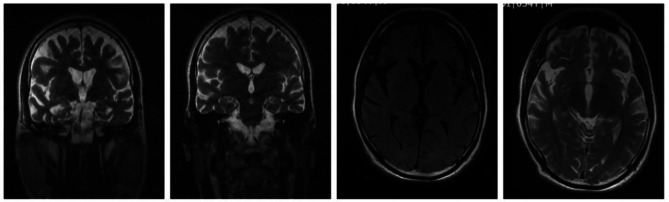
Brain magnetic resonance imaging (MRI): Frontotemporal lobar degeneration and cortical brain atrophy are more significant in the frontal, parietal and temporal lobes
